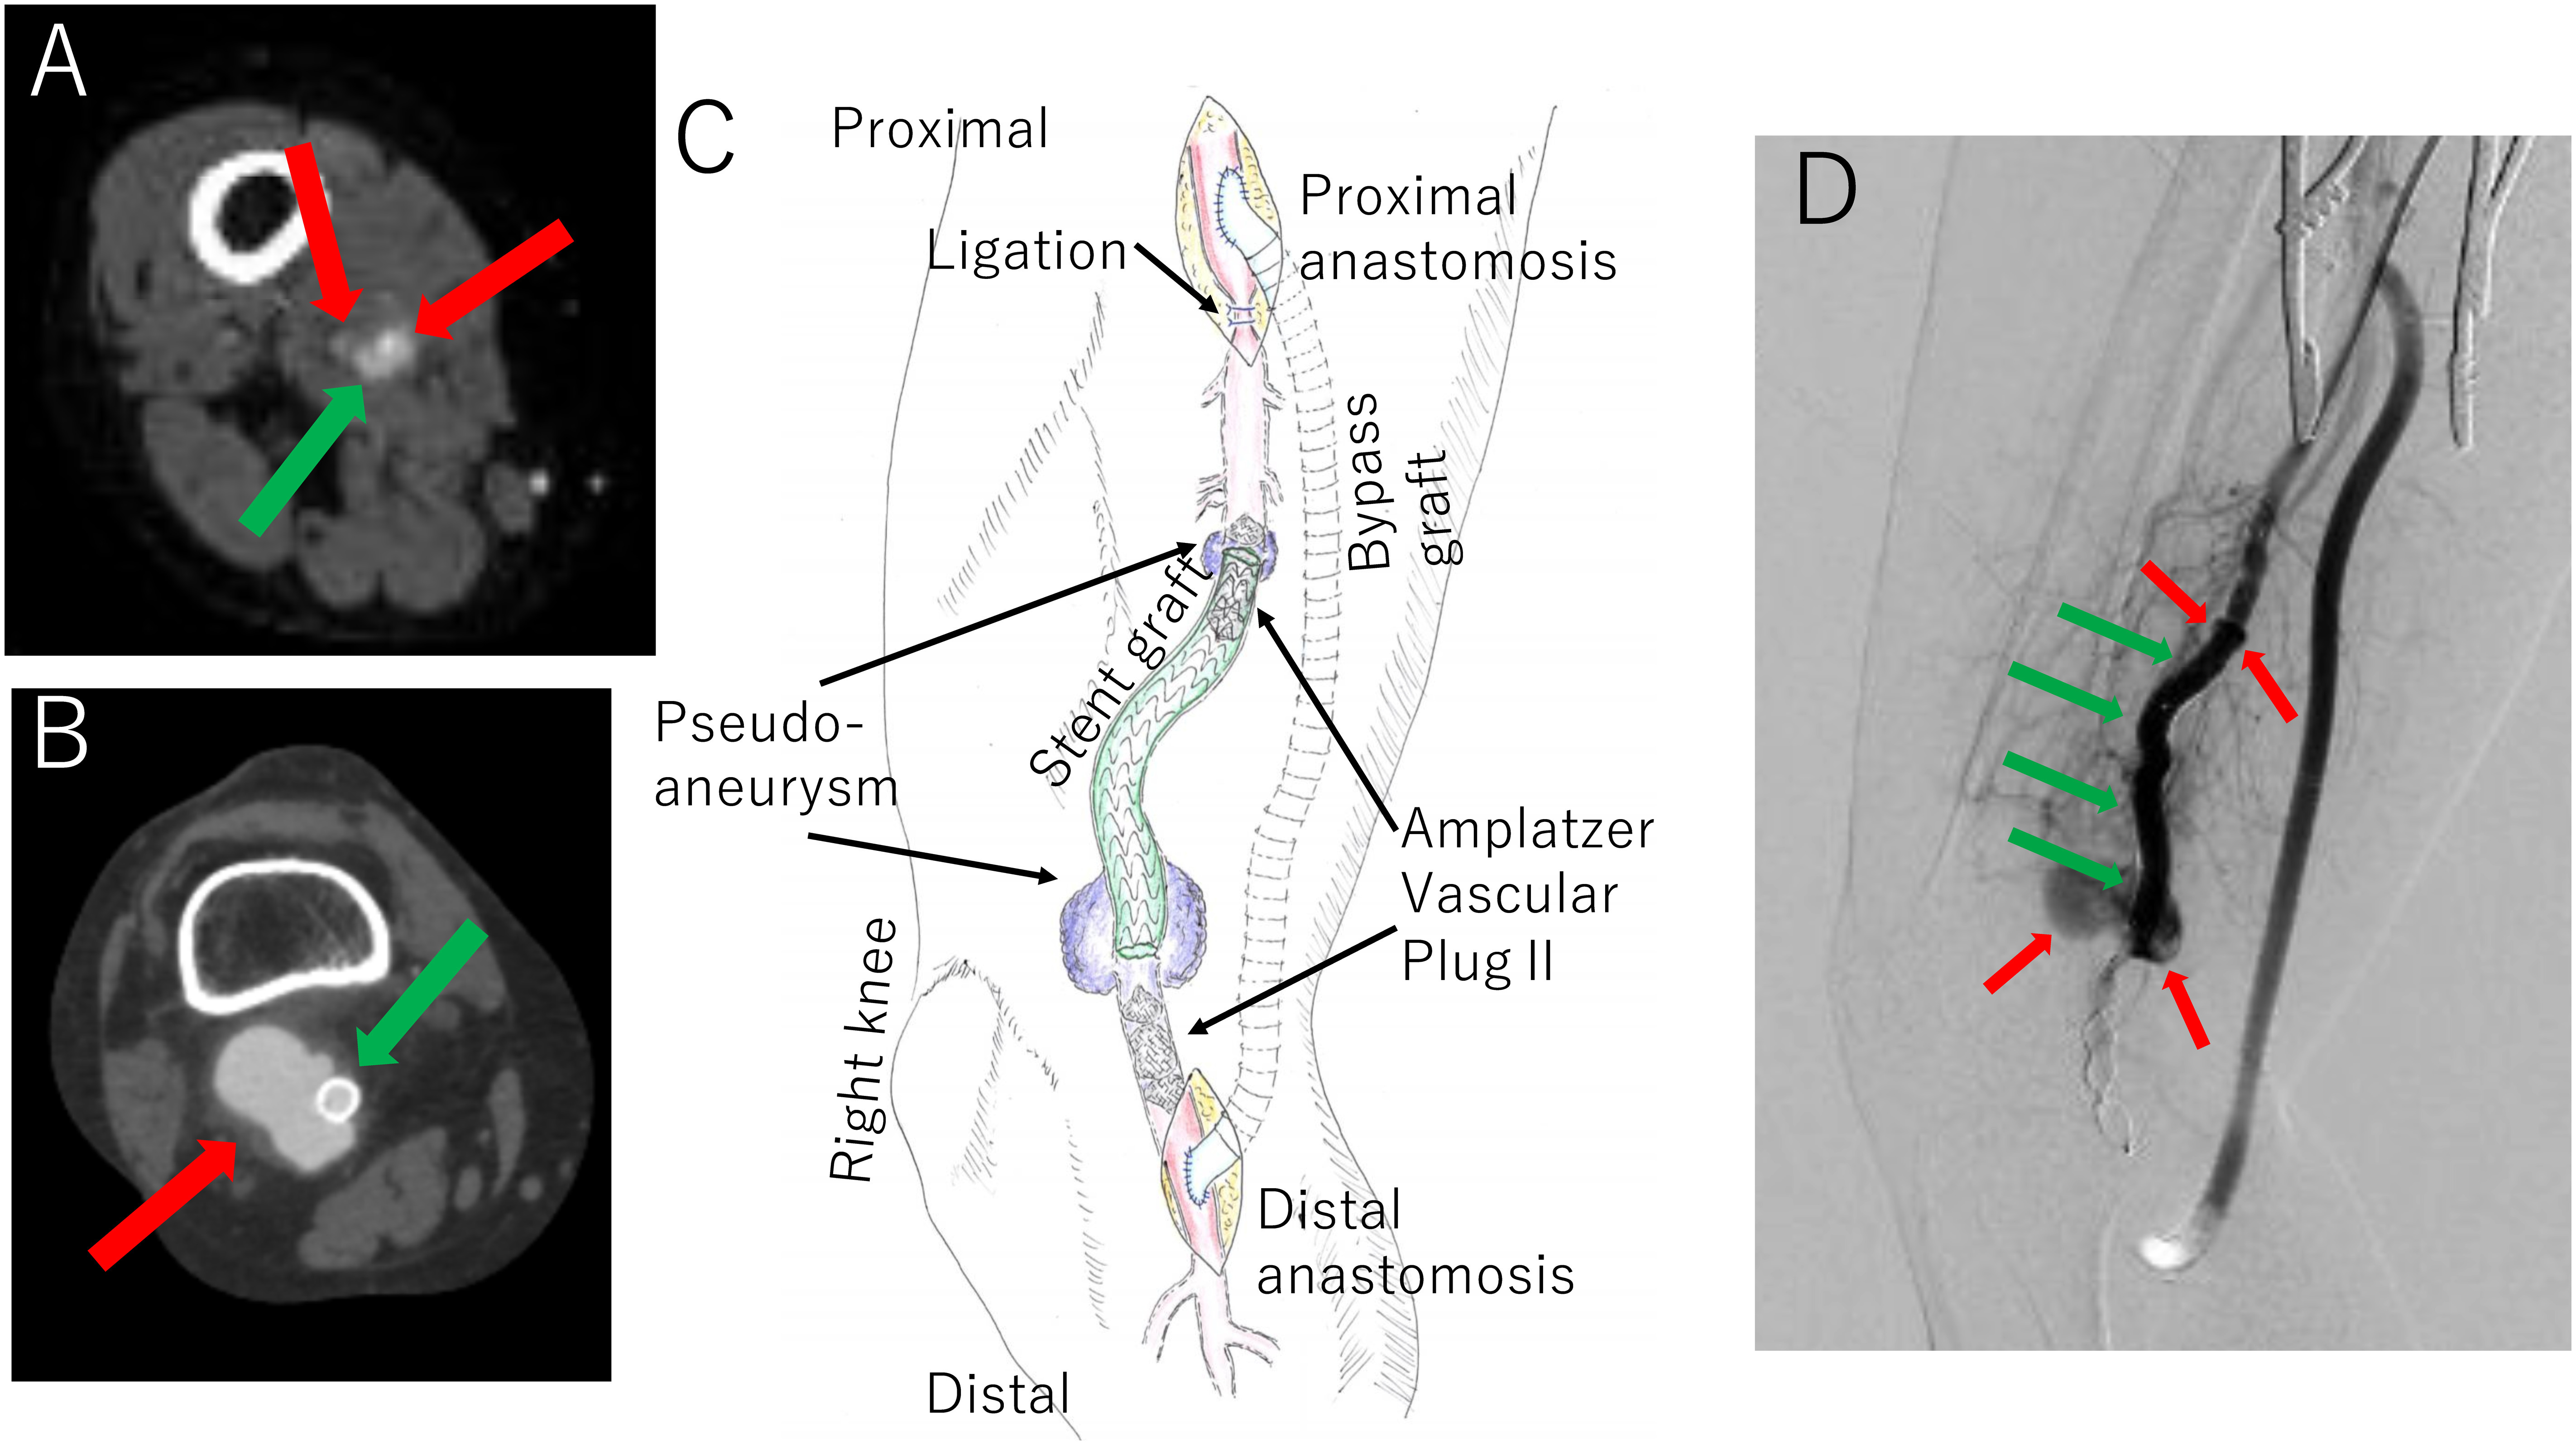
Fig. 2 Computed tomography images obtained on postoperative day 137 and the findings of exclusion and bypass procedures and embolization. (A) Axial view of the proximal edge of the stent graft. Green arrow, superficial femoral artery (SFA); red arrows, pseudoaneurysm. (B) Axial view of the peripheral edge of the stent graft. Green arrow, SFA with the inserted stent graft; red arrow, pseudoaneurysm. (C) Scheme of the surgery. (D) Digital subtraction angiography before ligation of the SFA. Green arrows, stent graft; red arrows, pseudoaneurysm.
